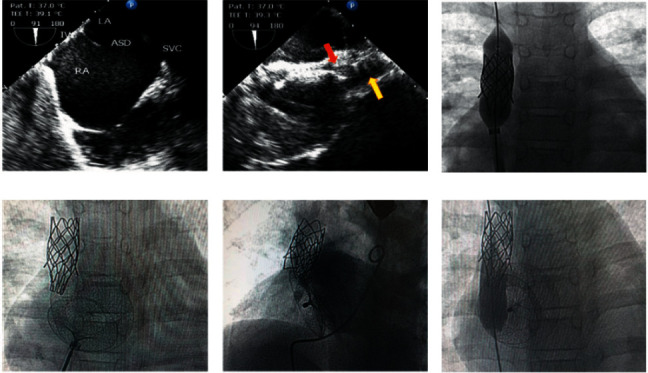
(a) Two-dimensional transesophageal echocardiography (TEE) imaging in long-axis bicaval view demonstrates a large secundum ASD with an absent rim near the SVC. (b) TEE in long axis view reveals the struts of the CP stent (yellow arrow) with the atrial septal occluder (red arrow) anchoring on the newly created superior rim by tight inflation of the covered-CP stent. (c) Cine imaging of the heart in the frontal projection demonstrates the inflation of the modified covered CP-stent 8 zig, 45 mm length by Z-med balloon size 22 × 40 mm. (d) Cine imaging of the heart in the frontal projection shows the deployed ASO in proper position with the projected part of the modified CP-stent out of RA disc before release. (e) Selective PA angiogram in four chamber view (LAO 35, cranial 35) demonstrates complete closure of the defect with no residual shunt. (f) Cine imaging of the heart in frontal projection demonstrates the inflated Z-med balloon (25 × 40 mm) slipped down in front of the septal occluder which did not capture the projected part of the modified CP stent, this balloon dilatation have been done three years later to achieve adult size of SVC.
